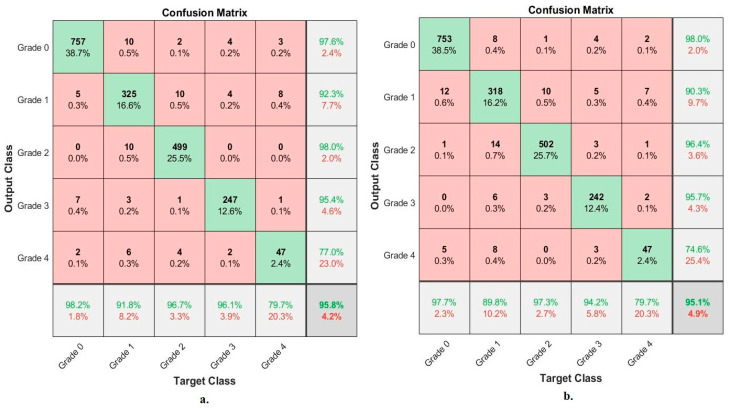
Display of the confusion matrix of the X-ray analysis of an OAI dataset for early diagnosis of severe KOA by FFNN with features of (a) VGG-19 (b) ResNet-101.
